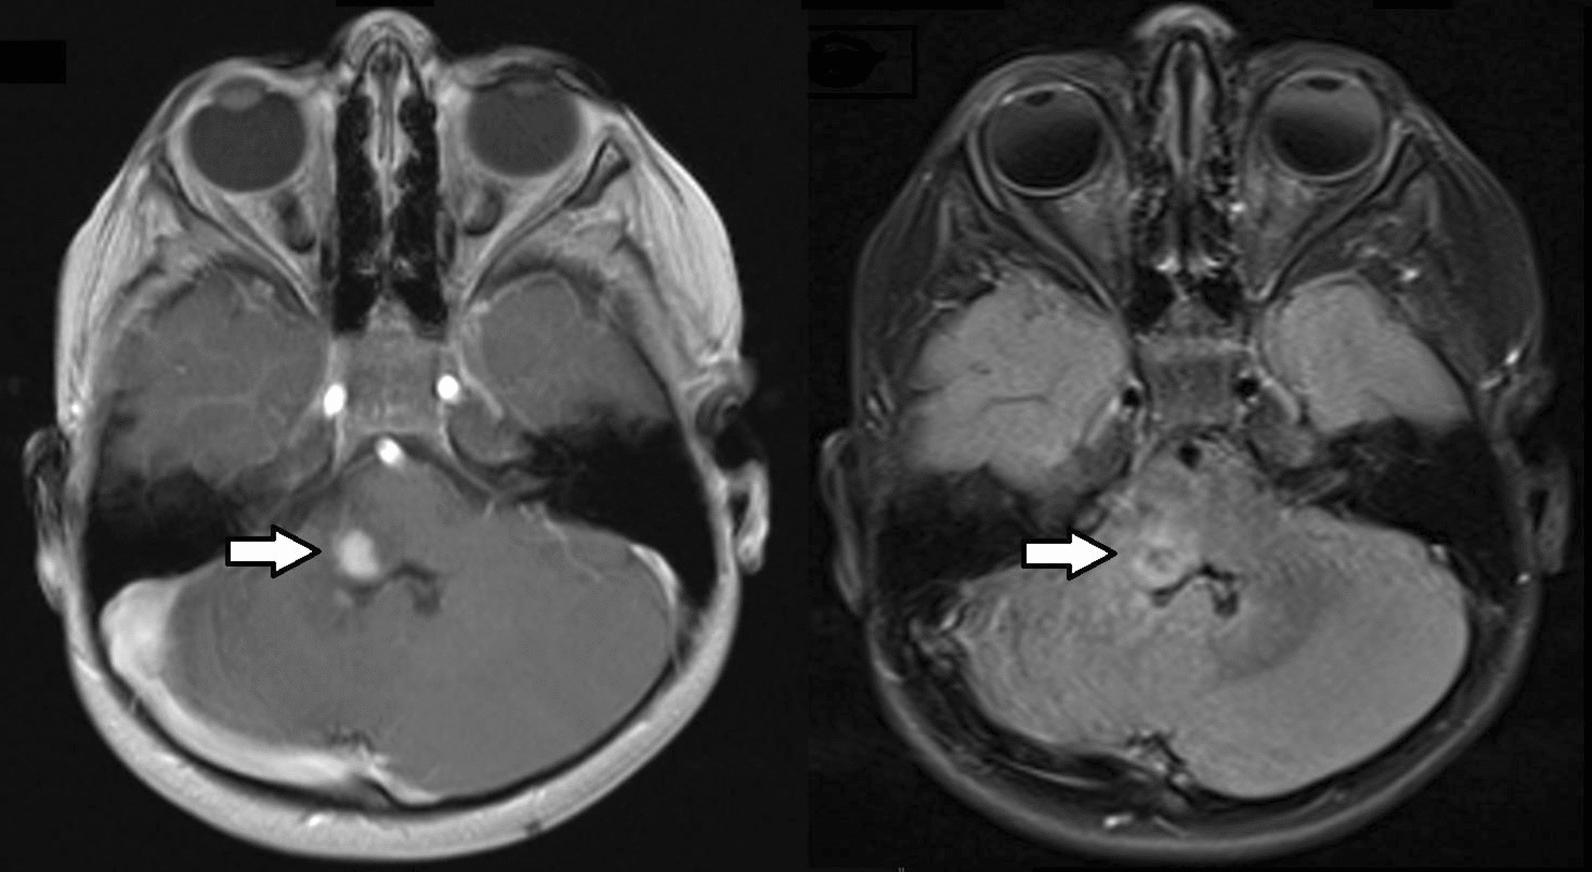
T1-weighted post-contrast (left panel) and T2/FLAIR-weighted (right panel) magnetic resonance images revealing a partially contrast-enhancing intra-parenchymal right medullary tumor (arrows). The tumor involved the right lateral aspect of the inferior pons, brachium pontis and ventral cerebellum
